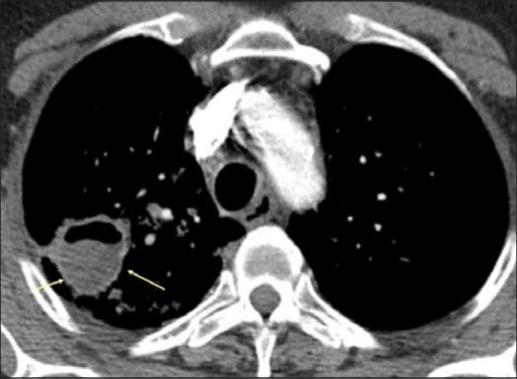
Tuberculosis.* This 68-year-old male previously lived in areas of endemic tuberculosis. He was admitted with productive cough and fever in the evening in the past two weeks. Now he has hemoptysis with a C-reactive protein level of 120 mg/L. The initial radiograph showed a cavity. CT with contrast showed a fluid-filled cavity in the upper right lung, with faint contrast enhancement in the wall.
